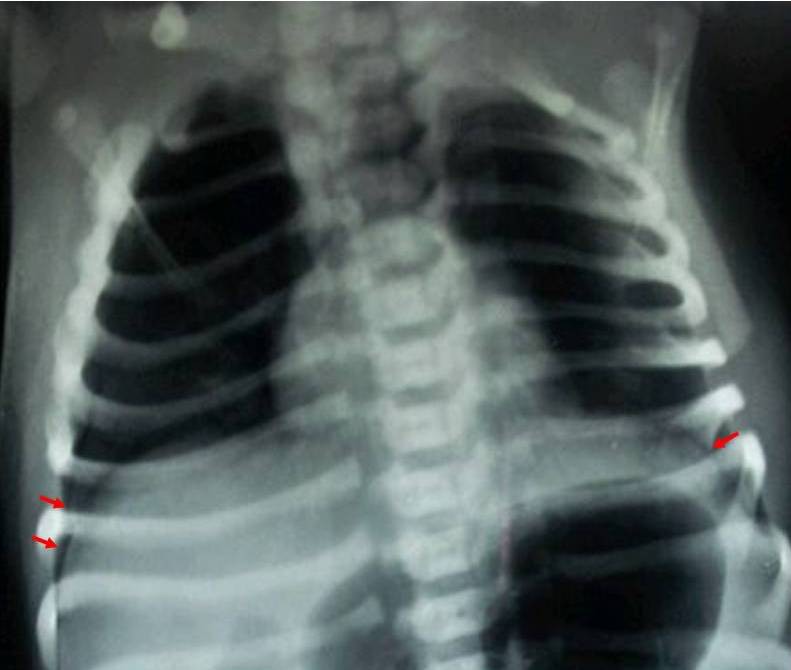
Figure 1:Chest X-ray (AP view) showing bilateral pneumothoraces
